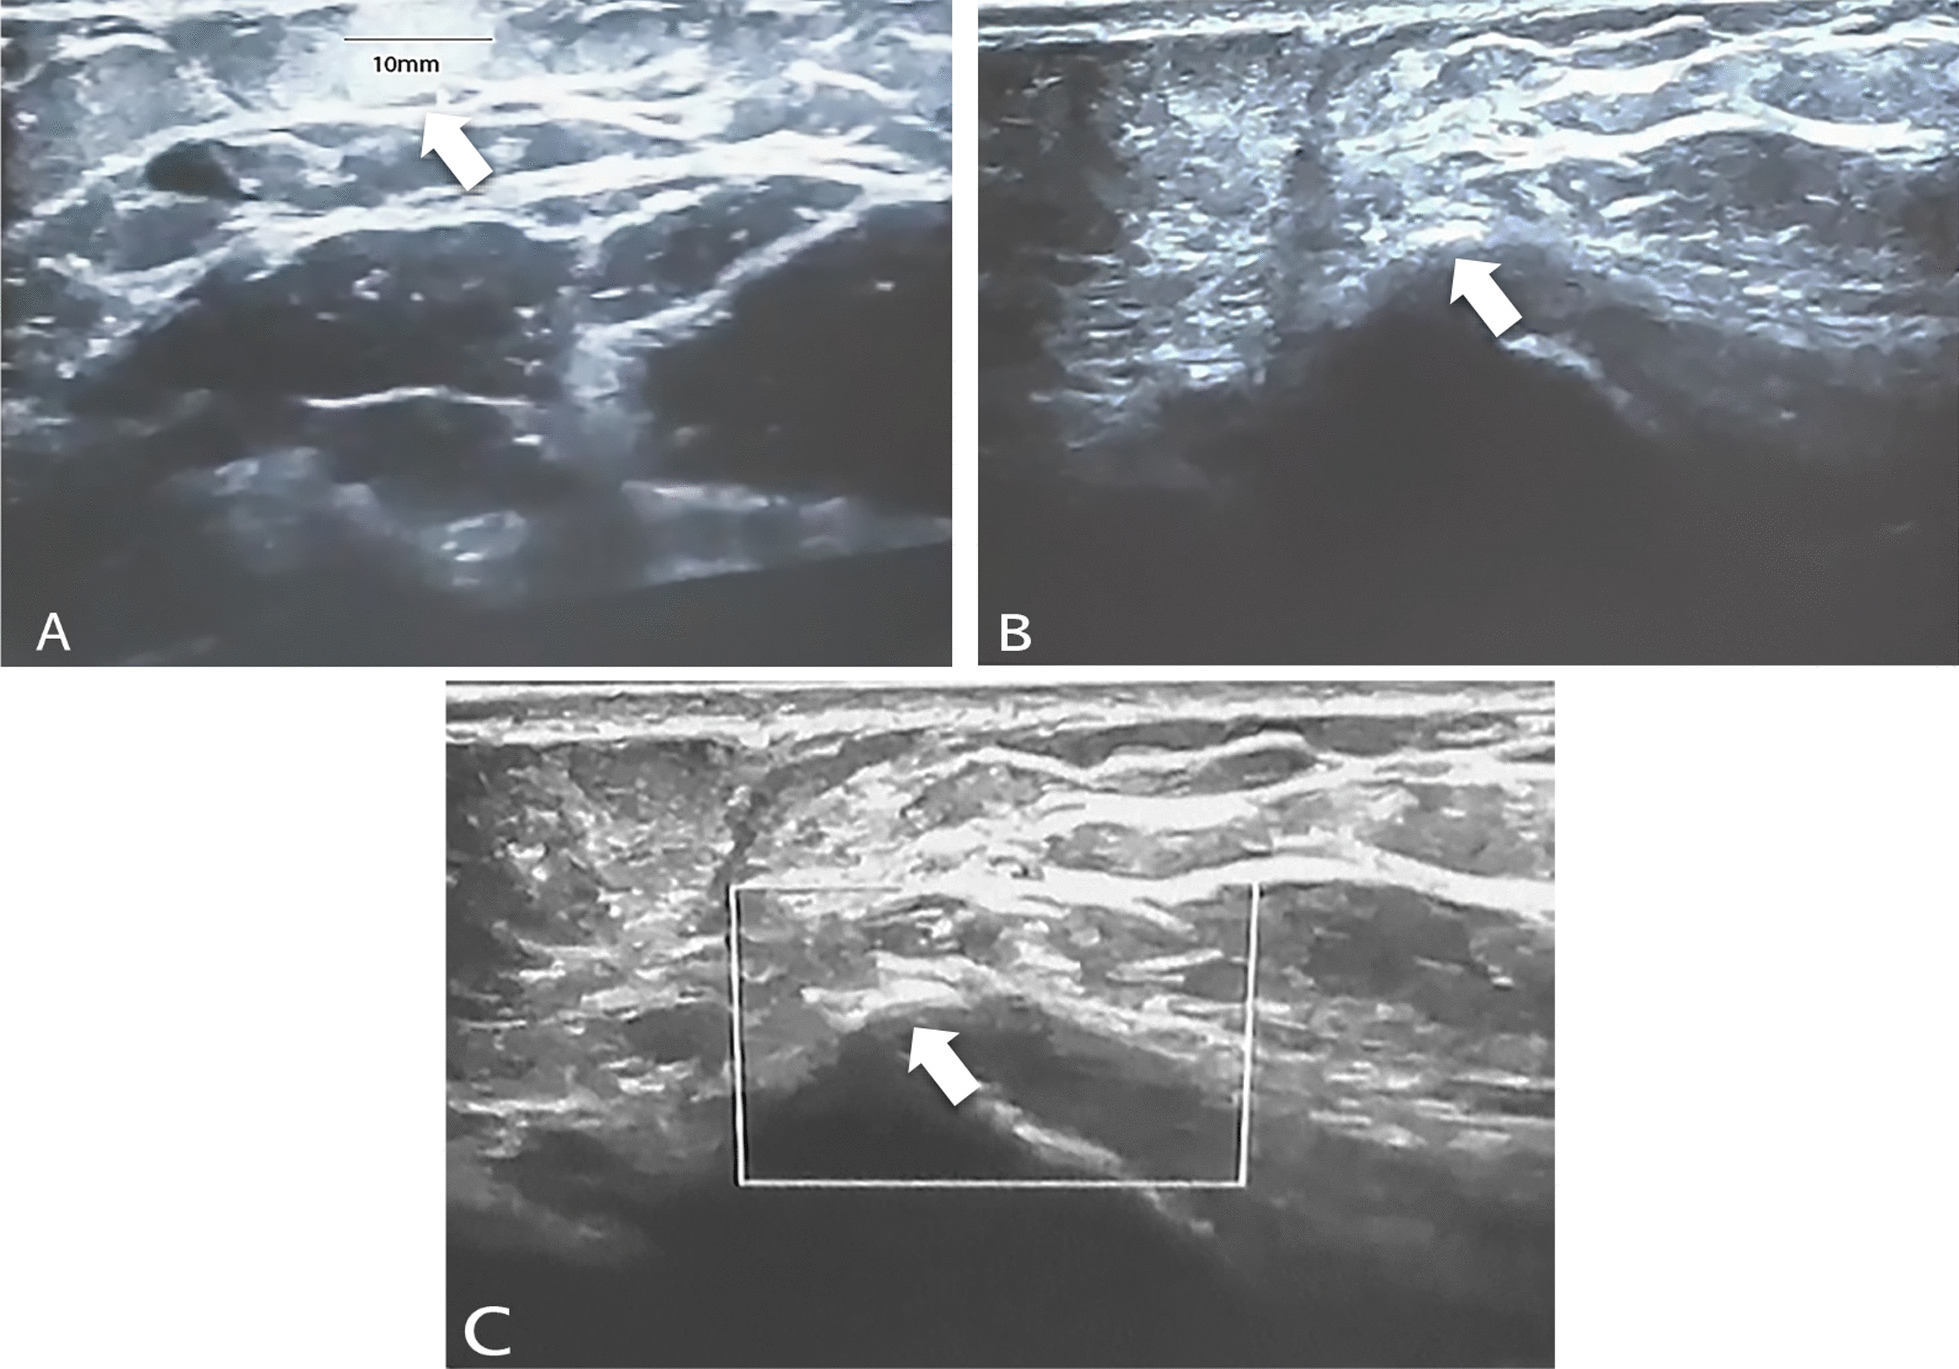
The ultra-sonographic imaging of patients with pain and a non-tender mass in the left elbow. A a well-defined, compressible hyperechoic lesion with a maximum diameter of 10 mm that is consistent with a subcutaneous lipoma (Arrow). B A US scan of the pronator teres tendon origin site revealed a thicker and more diffusely heterogeneous tendon with a dense hyperechoic calcification site, suggesting calcific tendinitis(Arrow). c On color Doppler ultrasound, there is no flow at the calcified site at the origin of the pronator teres tendon (Arrow)
